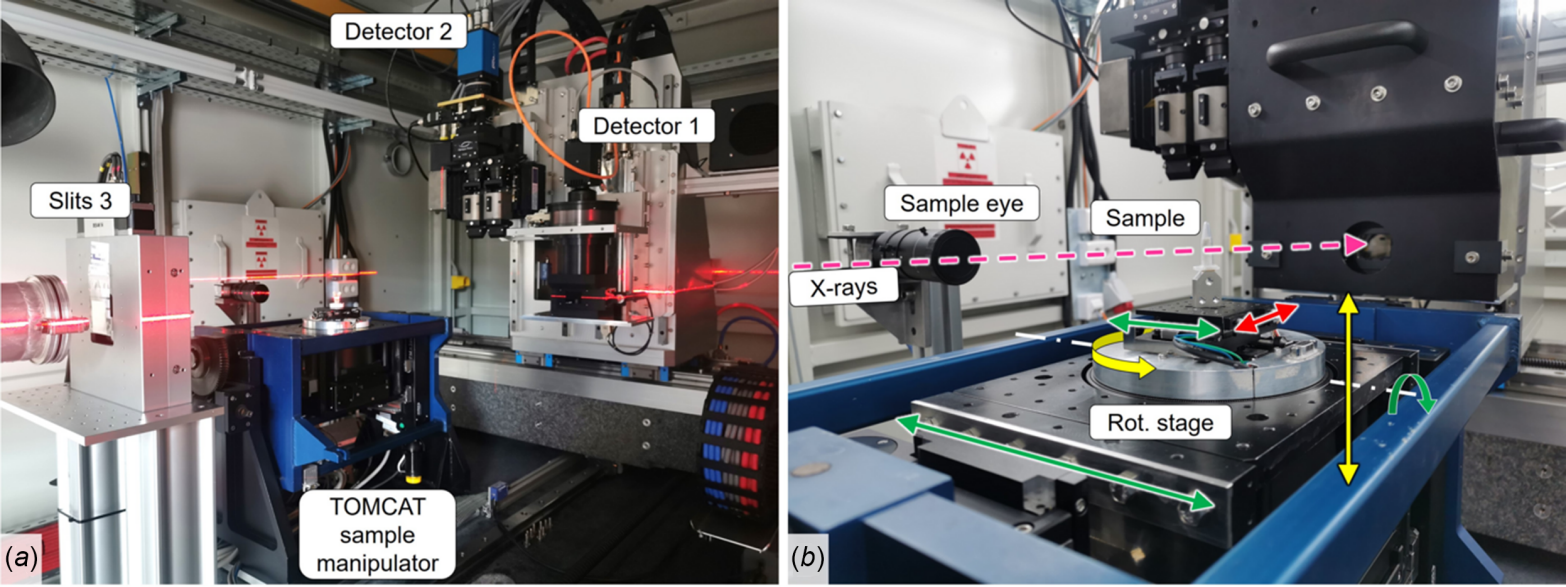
(*a*) BEATS X-ray radiography and tomography endstation with detectors 1 and 2 for white beam applications installed. For monochromatic beam experiments, detector 3 of Table 3 ▸ can be installed by replacing detector 2. A laser line helps the user in finding a preliminary sample alignment position. (*b*) Detail of the tomography sample manipulator indicating the axes of sample motion.
